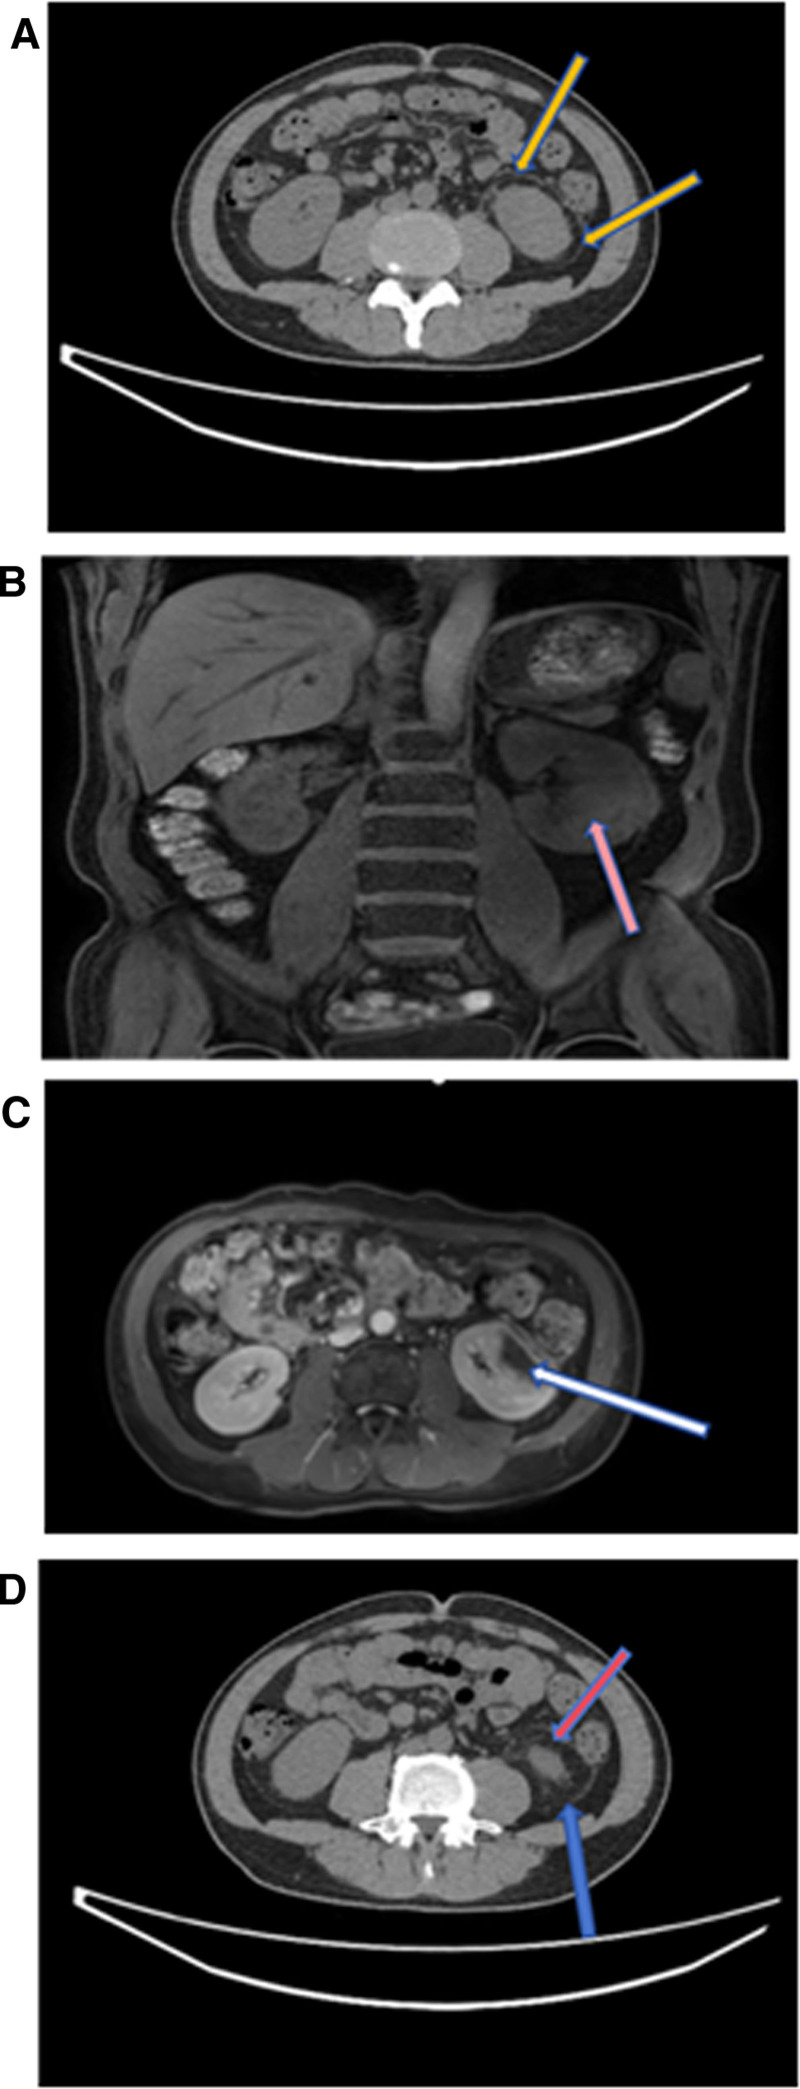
CT and MRI images (A–D). (A) The arrow points to an ill-defined, soft-tissue attenuation mass enveloping the left kidney (LK). Note the absence of a fluid-density center or rim enhancement, which are typical of an abscess. Instead, the infiltrative nature of the lesion is more consistent with retroperitoneal fibrosis or inflammatory infiltration. (B) Arrowheads highlight patchy areas of high T2 signal intensity within the left renal parenchyma and the perirenal mass. These represent sites of active inflammation or edema, which are characteristic of IgG4-RD. The black arrow indicates subtle stranding in the perirenal fat. (C) The arrow points to the extent of the infiltration process, showing the intermediate signal intensity on T1 weighted imaging. The lesion showed a mass effect, resulting in mild distortion of the renal contour. (D) Diffuse thickening and infiltration of the left perirenal fascia and Gerota fascia. Surrounded by fibroinflammatory tissue. This manifestation is a hallmark of IgG4 related retroperitoneal fibrosis. CT = computed tomography, IgG4-RD = immunoglobulin G4-related disease, IL = interleukin, MRI = magnetic resonance imaging.
